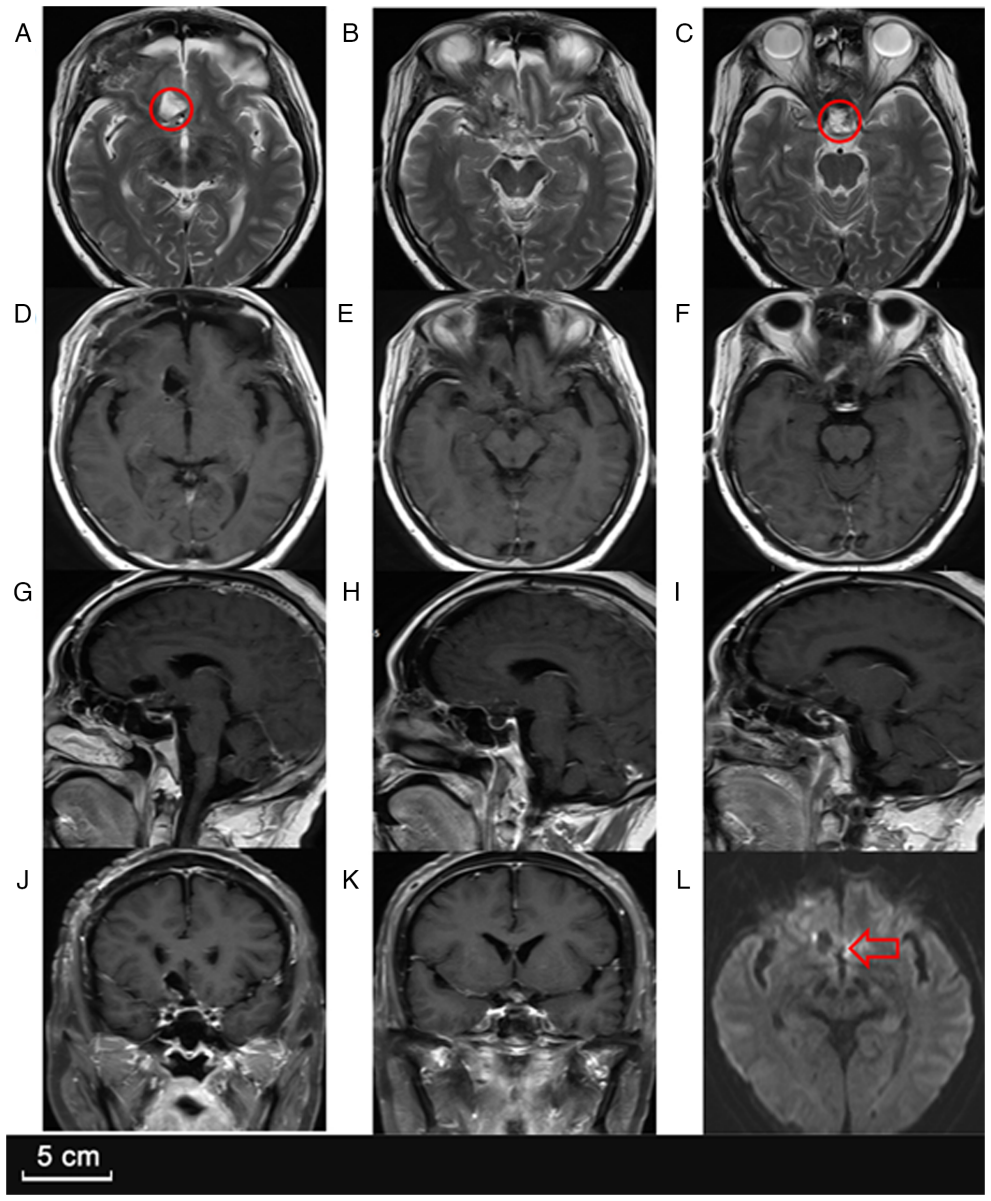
Postoperative MRI. (A-C) T2-weighted images showing high signal intensity in the (A) suprasellar (indicated by a circle) and (C) sellar (indicated by a circle) regions, consistent with cerebrospinal fluid. (D-K) Contrast-enhanced T1-weighted images showing no marked enhancement in the operative area. (L) Diffusion-weighted images indicating that the lesion had been completely removed and the pus had been drained (indicated by the arrow).
